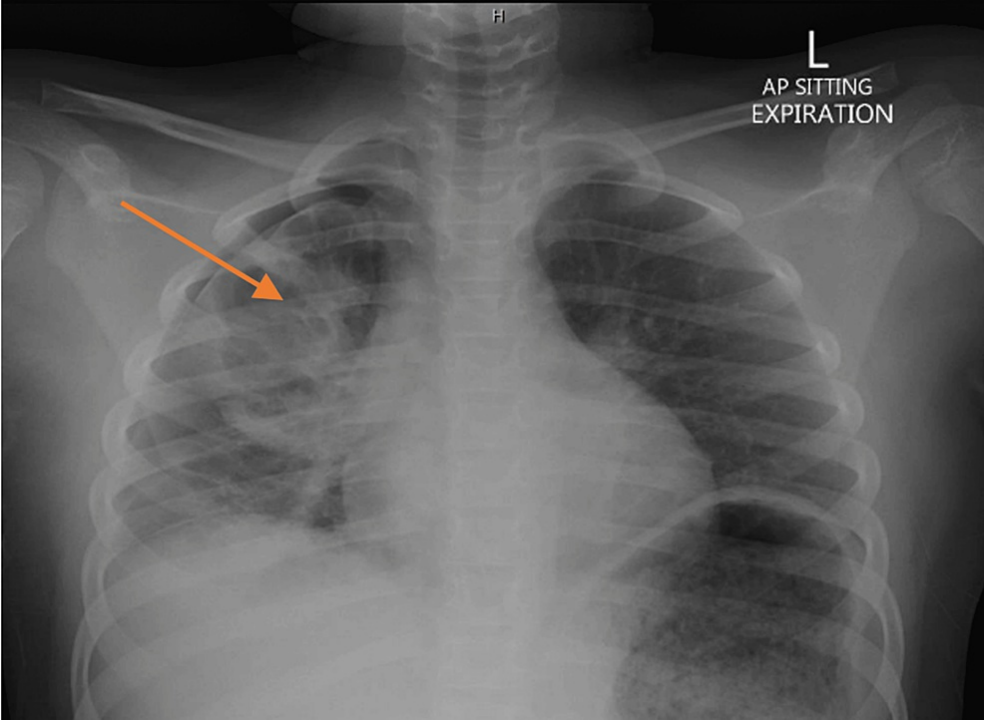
Chest X-ray. Small right-sided pneumothorax and right upper zone opacity. The arrow pointing to the right upper zone opacity.
